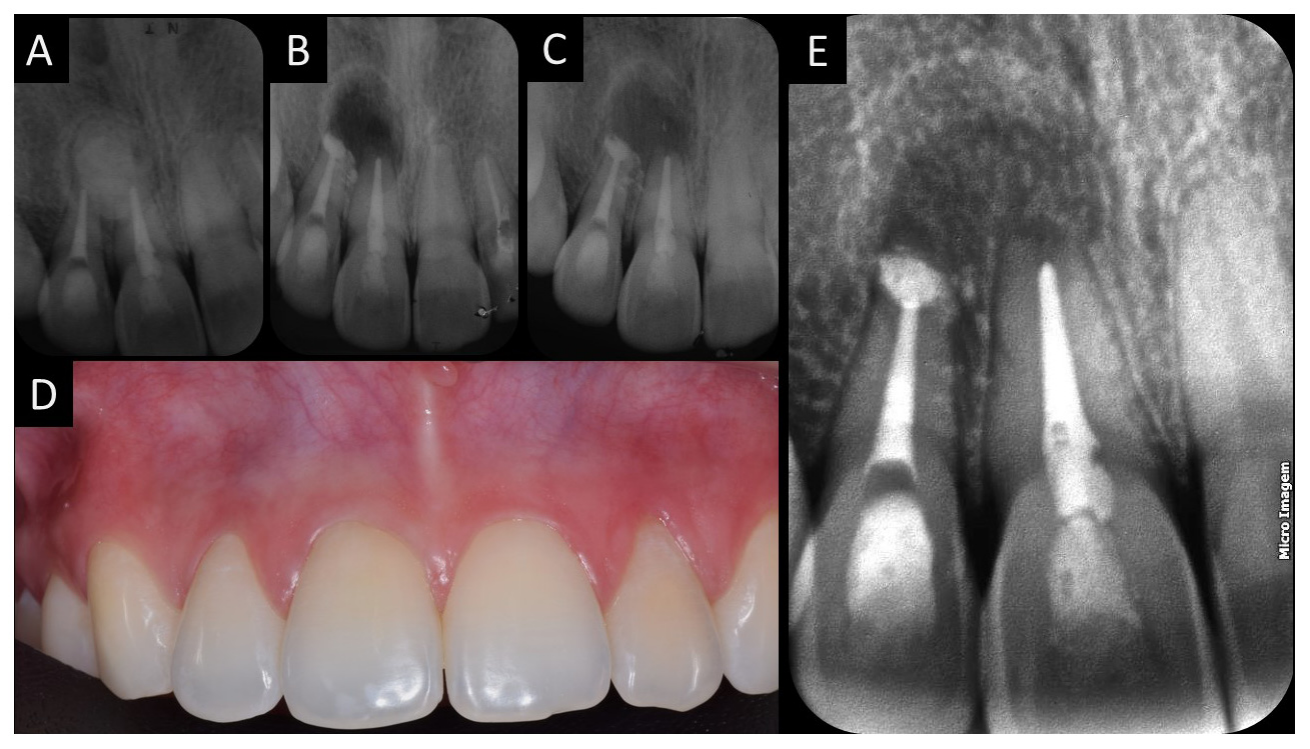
Radiographic preservation. A) Periapical radiography of the endodontic treatment before surgery;
